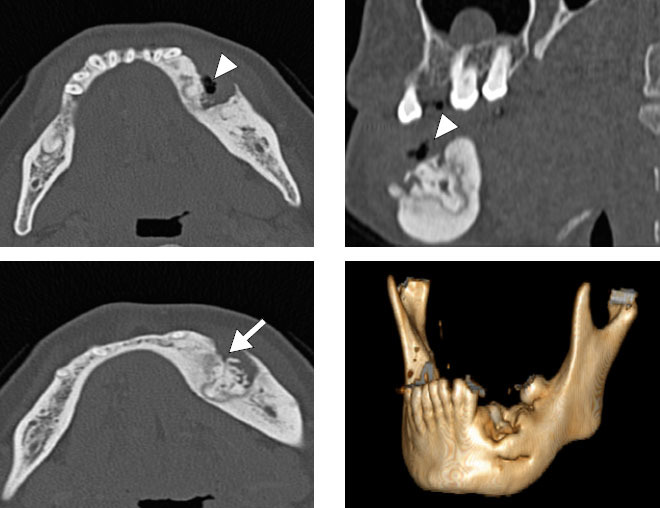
A 53-year-old male patient with mandibular osteomyelitis. Axial CT
